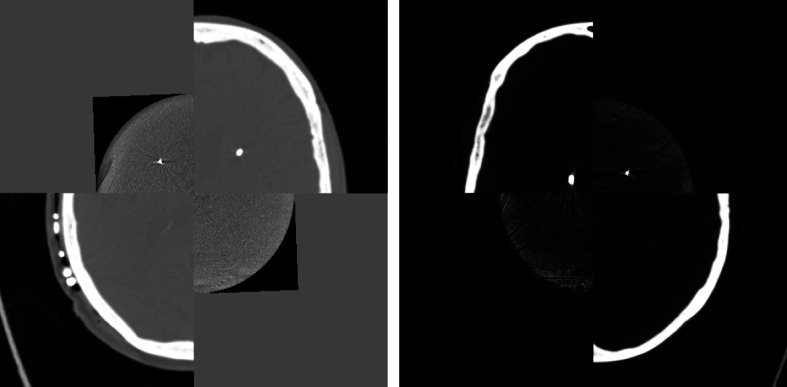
An example of CT weighted DynaCT images registered using mutual information. The two registered images are shown interleaved in a checkerboard pattern
